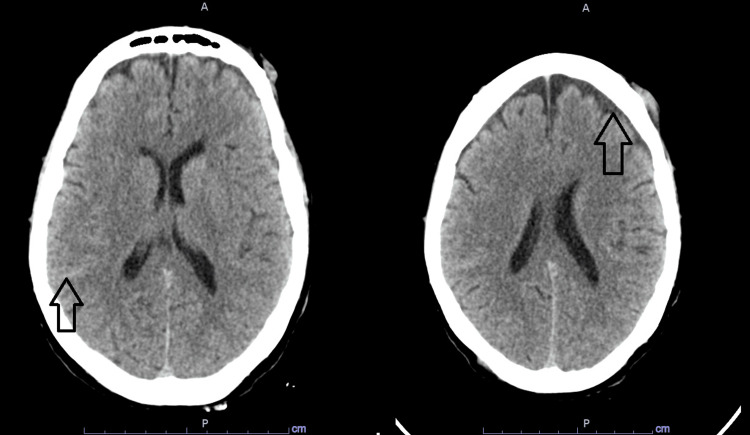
CT Head CT head showing mixed density left-sided frontal subdural hematoma (arrow on the right) and hyperdensity in the right posterior sulci suggestive of subarachnoid hemorrhage (arrow on the left).
